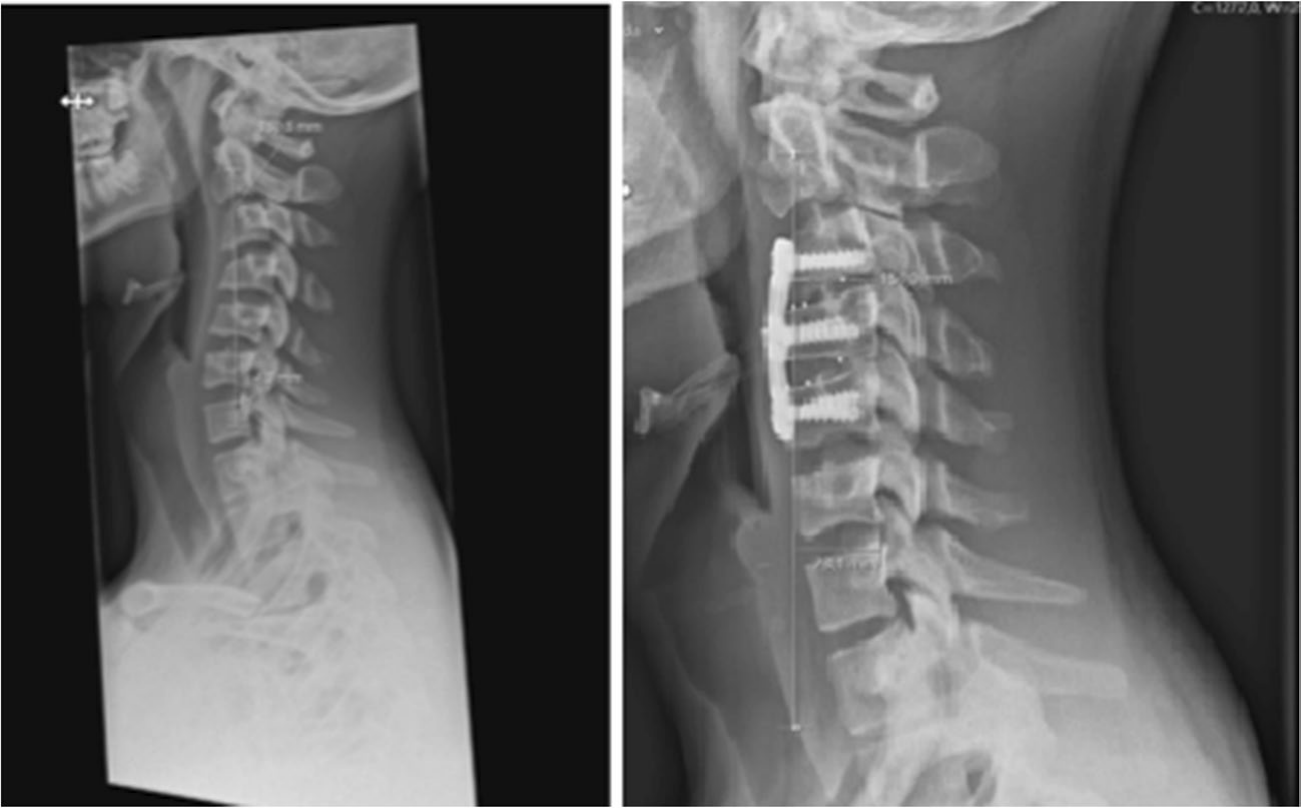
Pre and postoperative X-ray after surgical treatment with anterior cervical discectomy and fusion C3–C5. The cCVA before surgery was 4.3 mm and 23.1 mm after surgery. There was a clear reduction in the Ishihara index at the latest follow-up but with changes in alignment in the subaxial spine leading to changes in the cSVA (from 3 to 27 mm) (Table 1)
